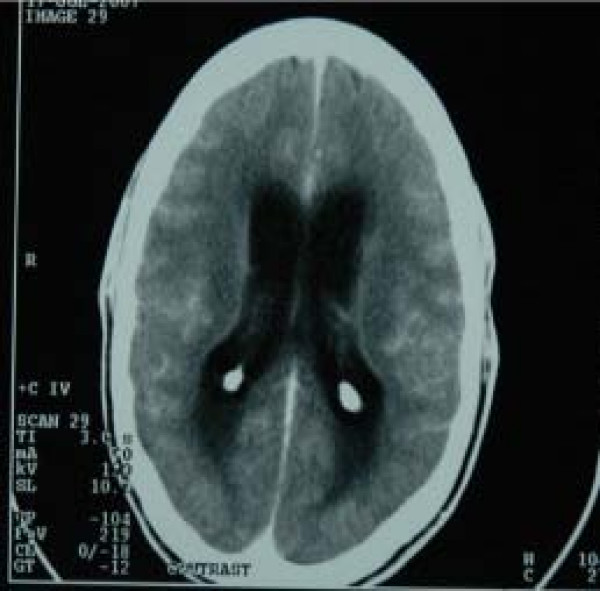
Brain computed tomography scan of case 2 showing dilated ventricles and calcification of choroids plexus.
